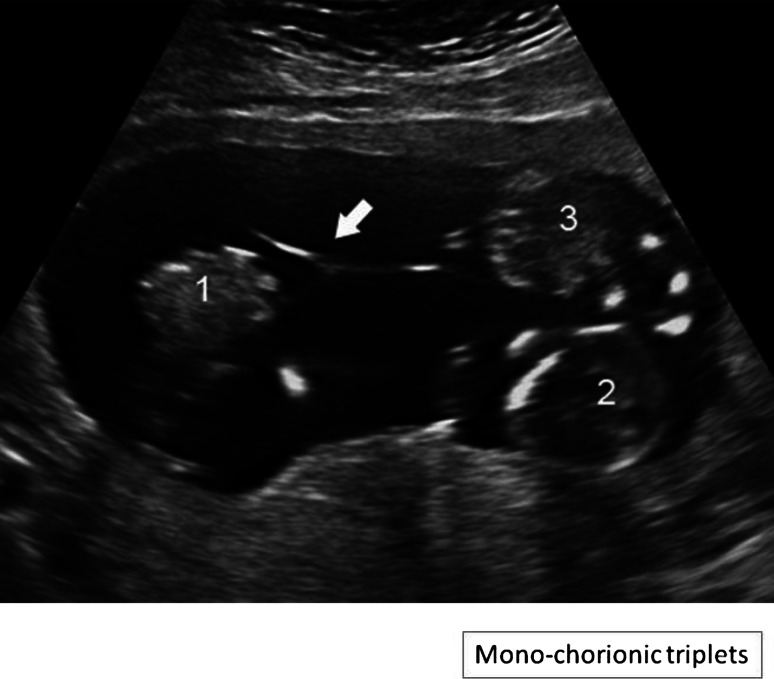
Ultrasound picture of a monochorionic, and therefore monozygotic trio at 12 weeks gestational age. The *arrow* indicates the meeting pointing point of three amniotic membranes. Numbers indicate the three fetuses. (Used with permission from Lamb et al. 2012)
